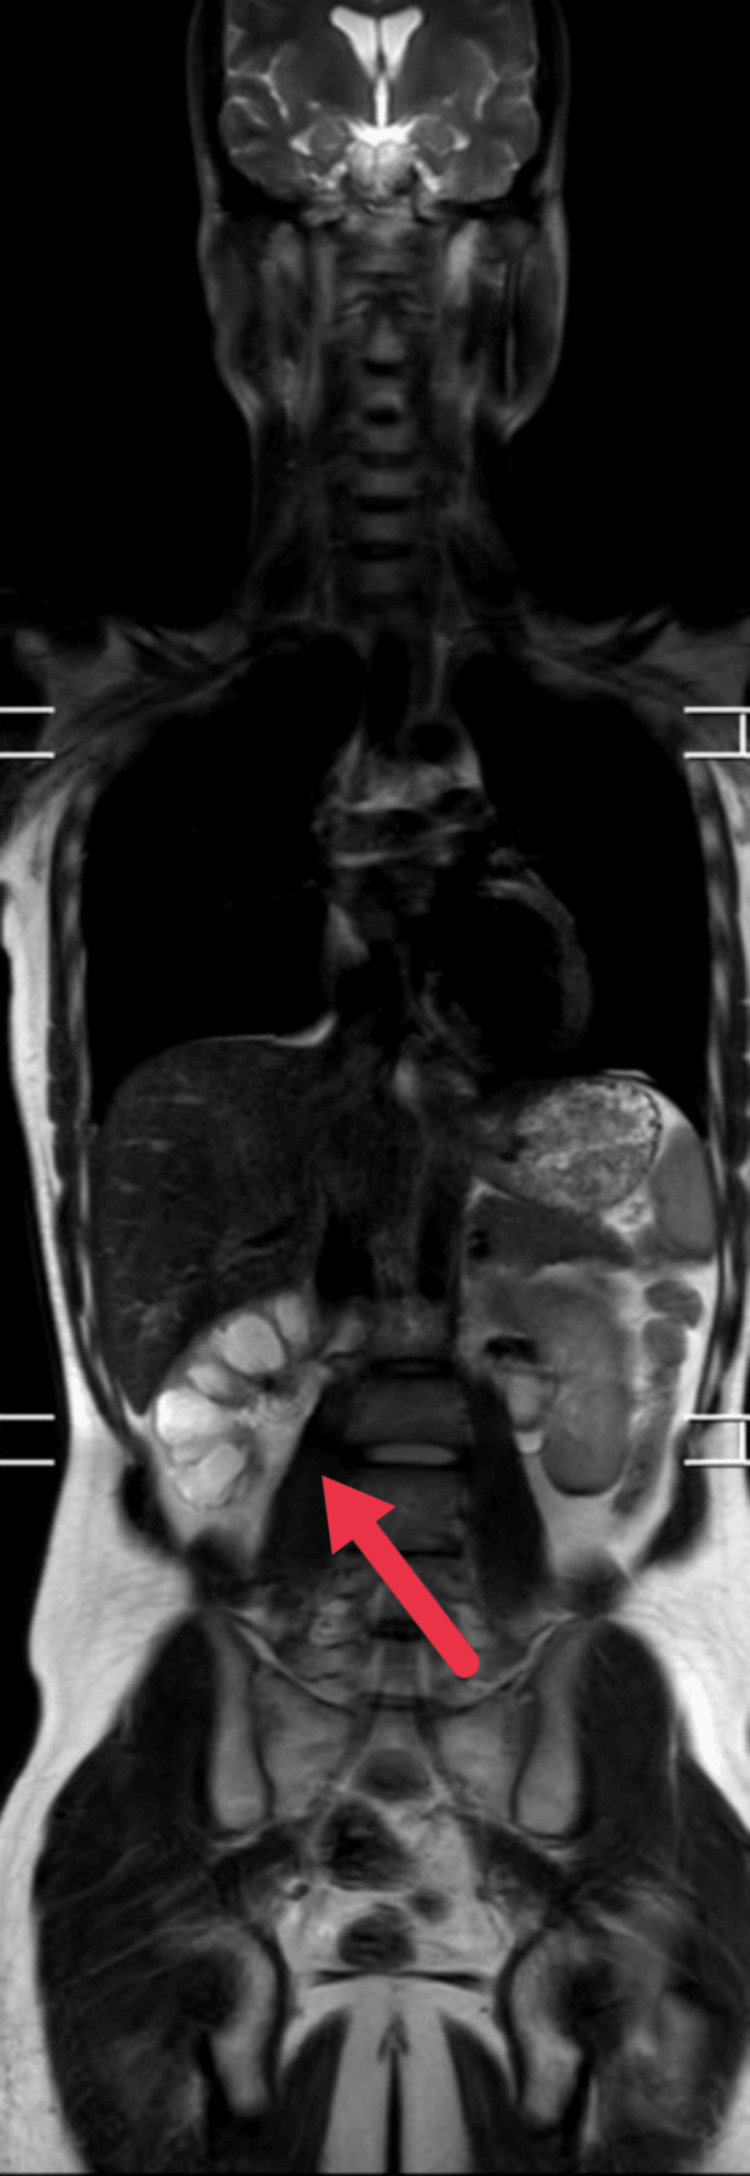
MRI showing grade 5 hydronephrosis MRI: magnetic resonance imaging
